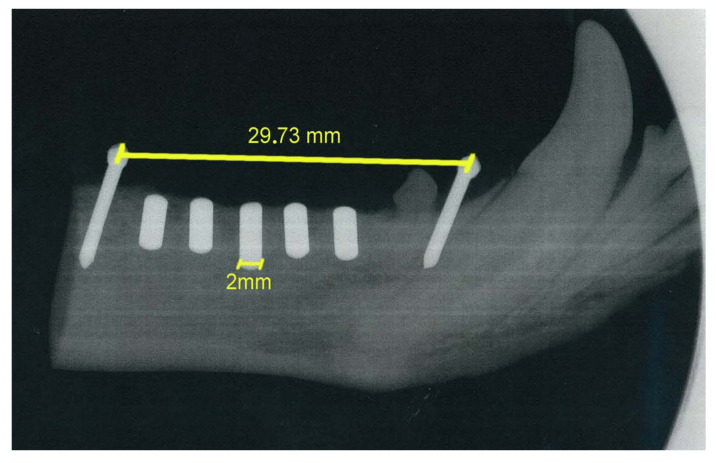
Digital radiograph of mandibular block with implanted cylinders.
